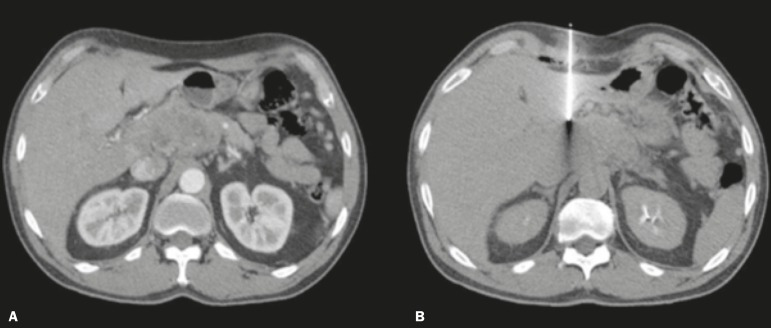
CT-guided percutaneous biopsy using the trans-organ approach.
